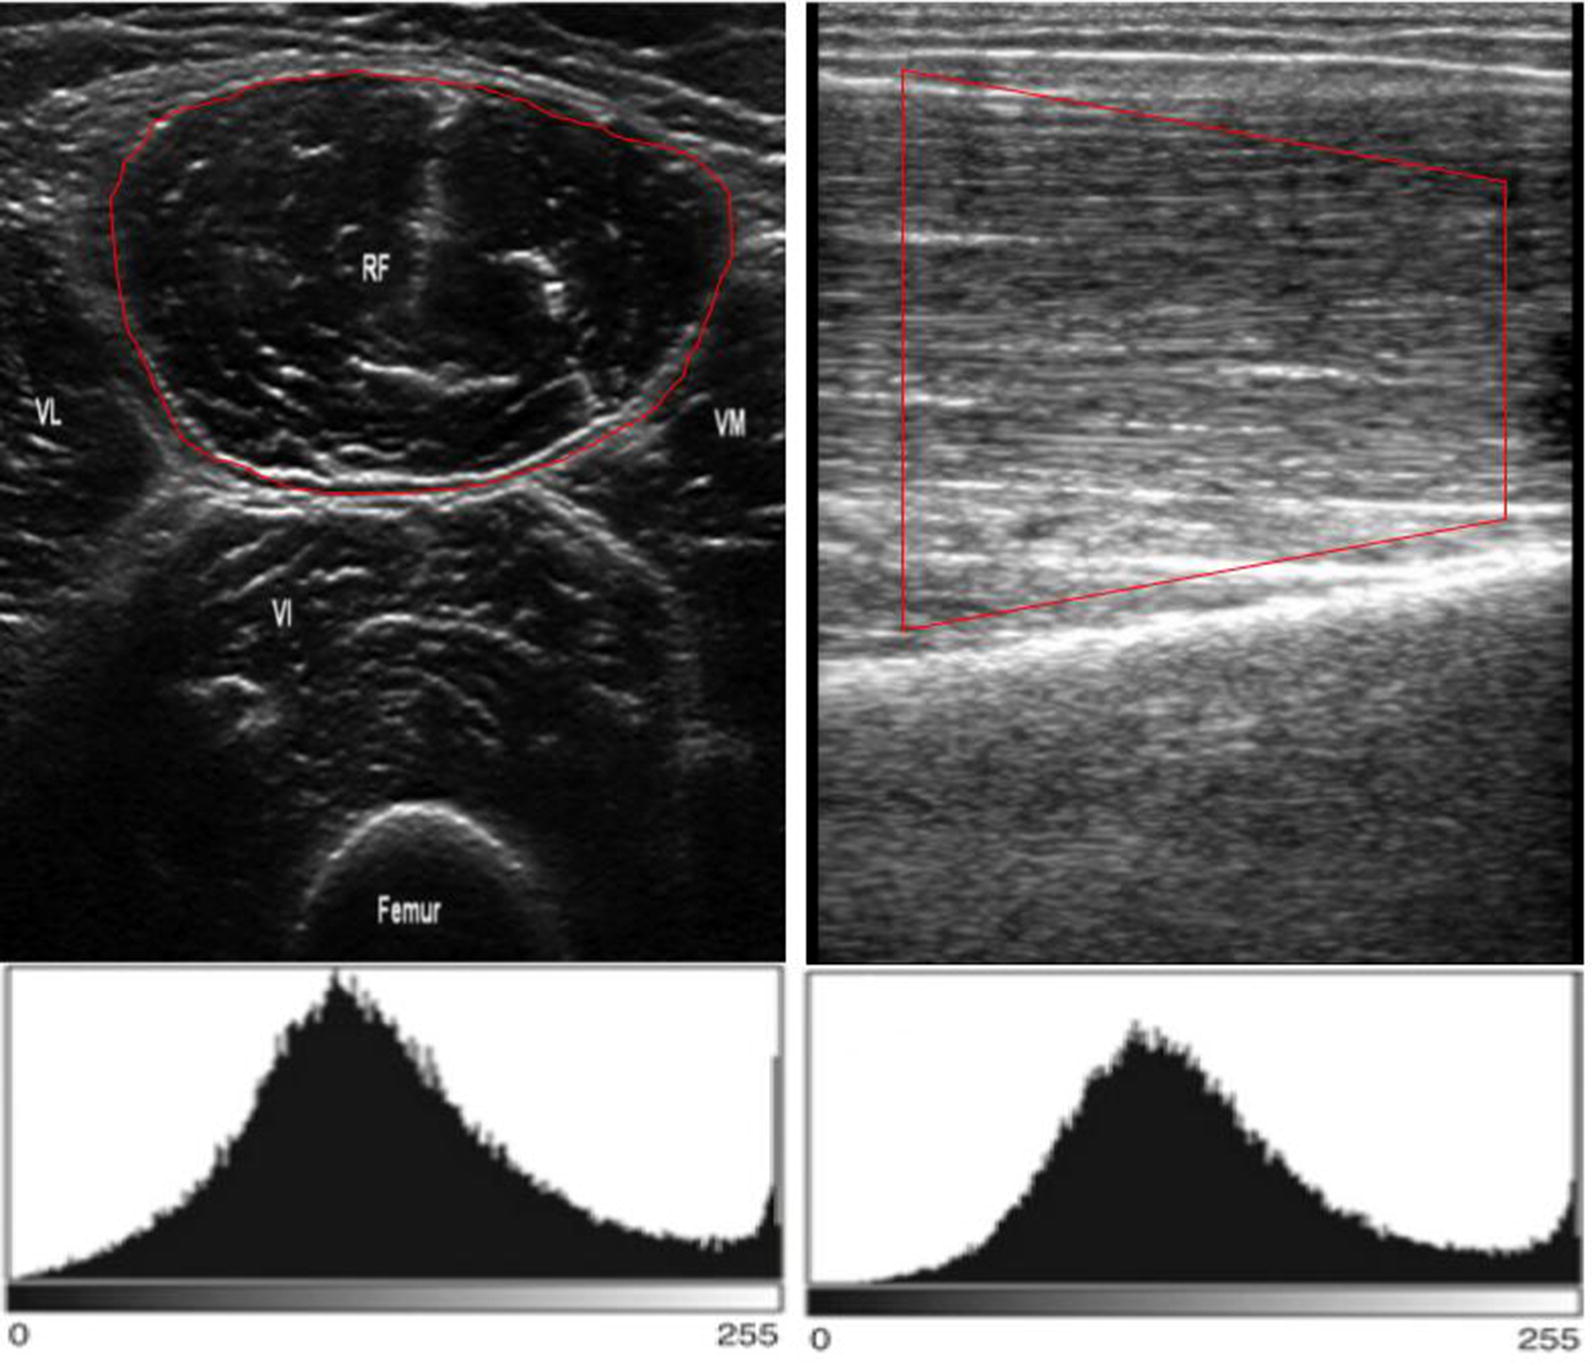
The muscle ultrasound echointensity. An example of the grayscale histogram in the transverse (right) and longitudinal (left) axis of the rectus femoris
